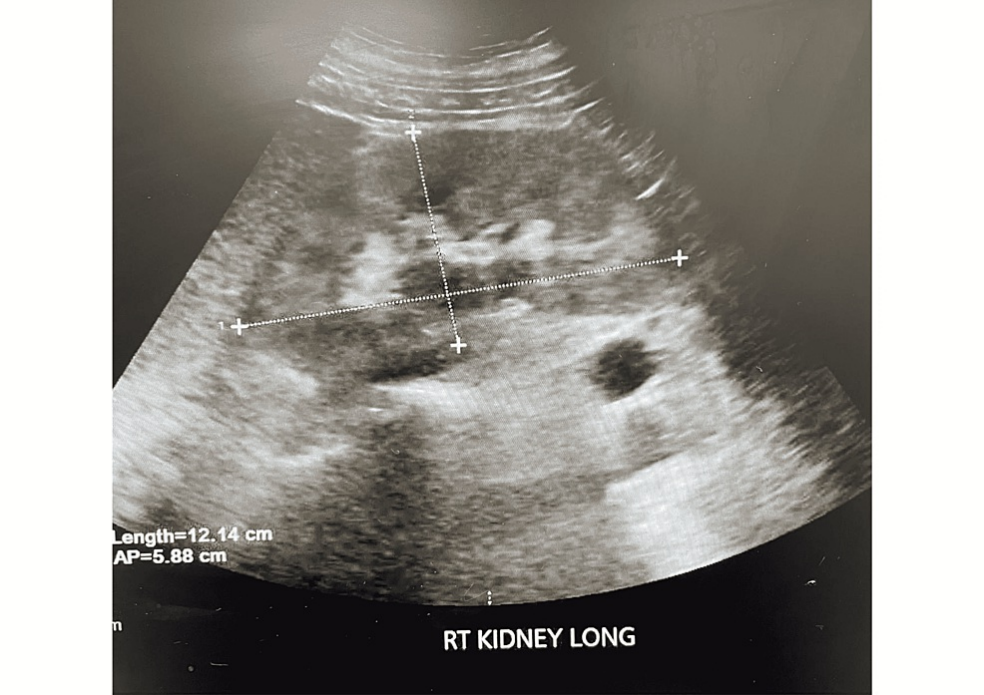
Ultrasound of right kidney showing diffuse increased echogenicity of renal parenchyma consistent with chronic renal disease.
